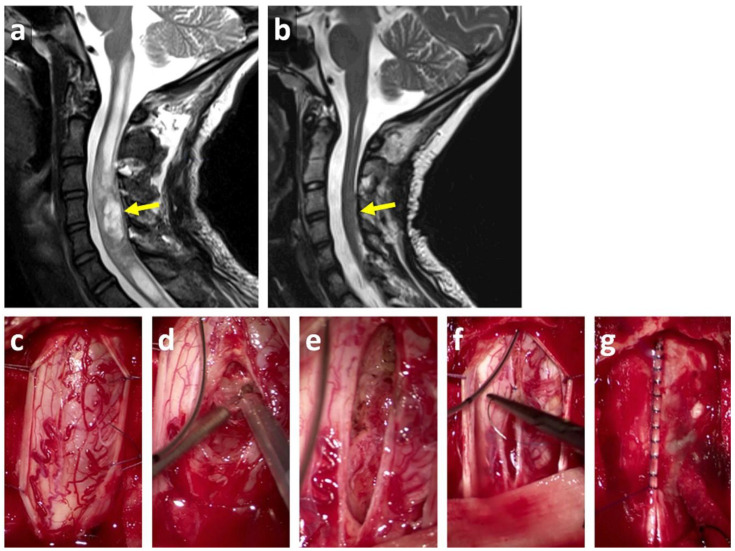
MRI presentation of a cervical pilocytic astrocytoma grade I of the World Health Organization (WHO) grading system (yellow arrow) before (a) and after surgical resection (b) in a 14-year old girl. Surgical situs shows opening of the dura (c), CUSA (cavitronic ultrasonic surgical aspirator) de-bulking (d), opened cavitying of the spinal cord with partially removed tumor (e), closure of the spinal cord (f) and dural closure (g).
